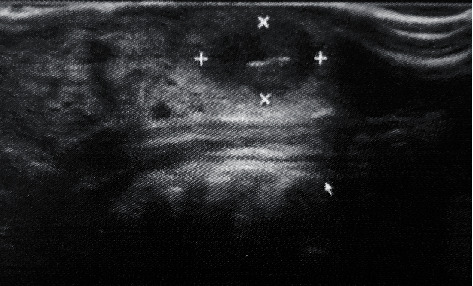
Ultrasonography showing a circumscribed nodular lesion. The echographic aspect was suggestive of a foreign body inside, suggesting an image compatible with a thorn, with granuloma formation.
